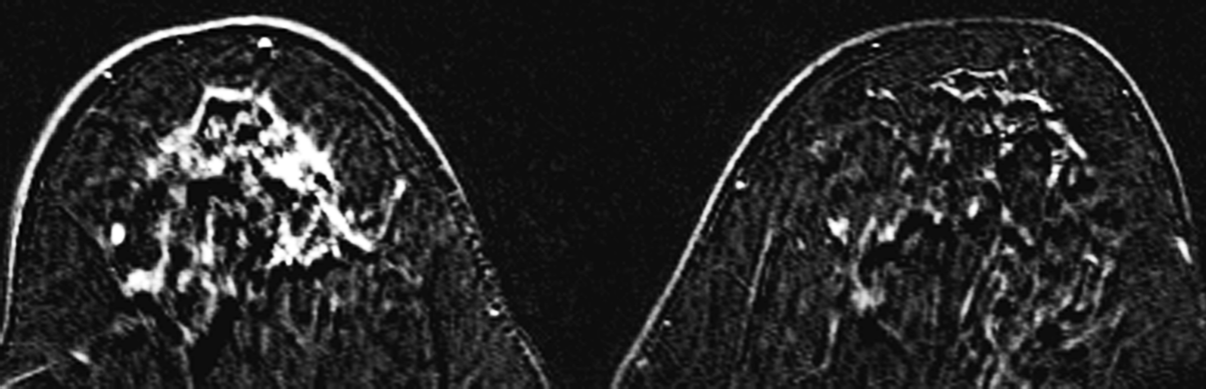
MRI. MRI demonstrates a large, irregular, enhancing mass with spiculated margins in the right breast. Pathology showed invasive lobular carcinoma.
